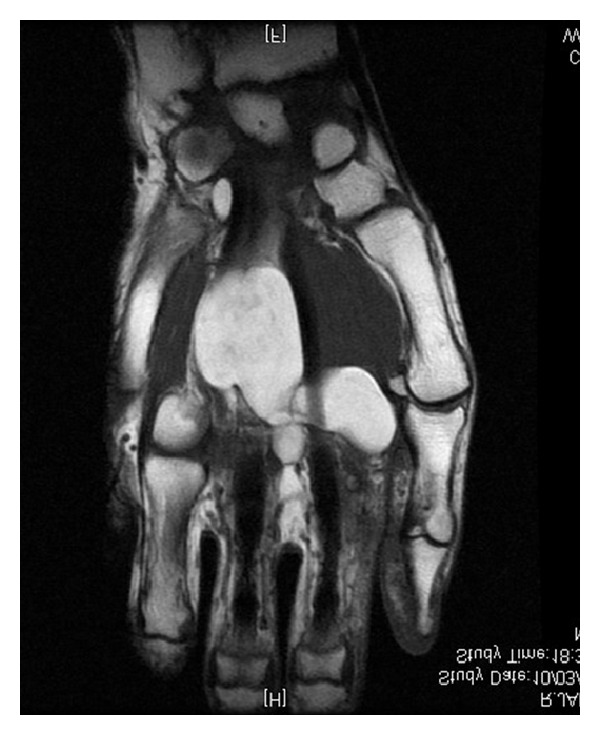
MRI frontal view showing tumor's infiltration without invasion.
